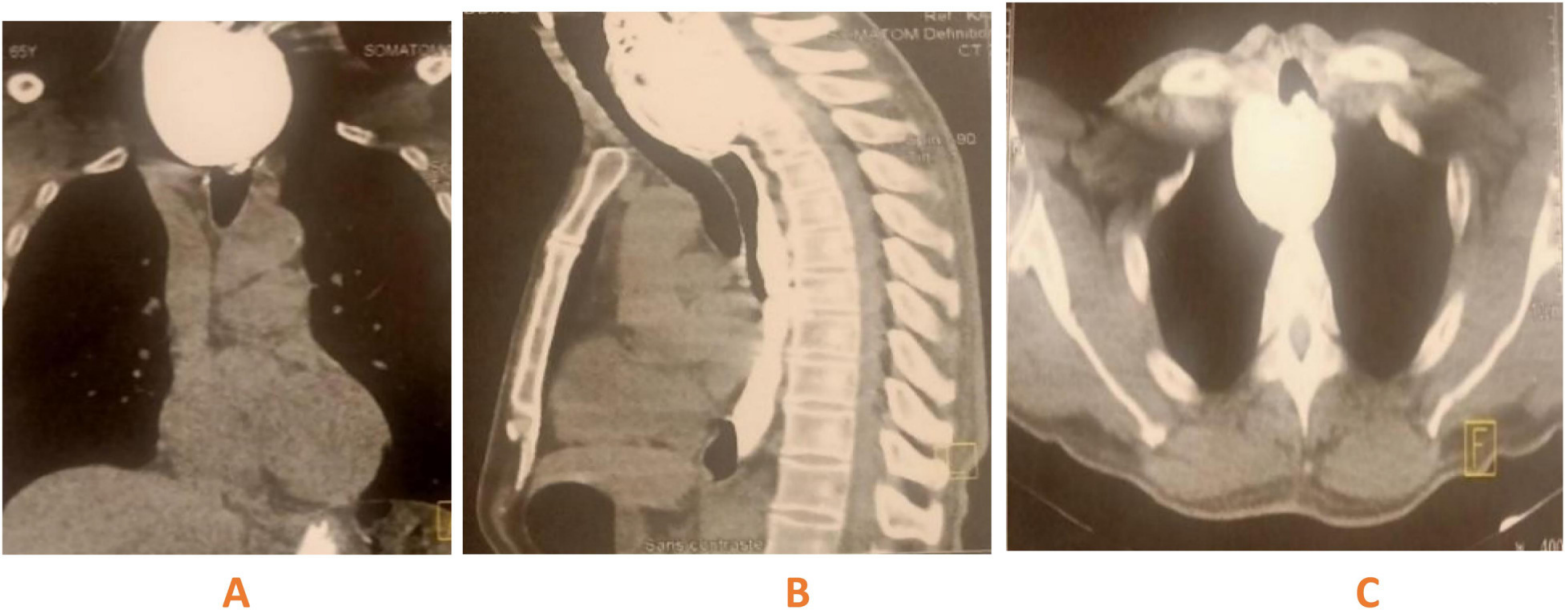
Cervicothoracic CT imaging after oral contrast (A: coronal, B: sagittal, C: axial) reveals a sizable retropharyngeal air-fluid collection measuring 7.2 × 3 cm, corresponding to a diverticular pouch situated anterior to Killian's triangle. The pouch causes anterior displacement of the neck's visceral axis and posterior compression of the esophageal wall.
